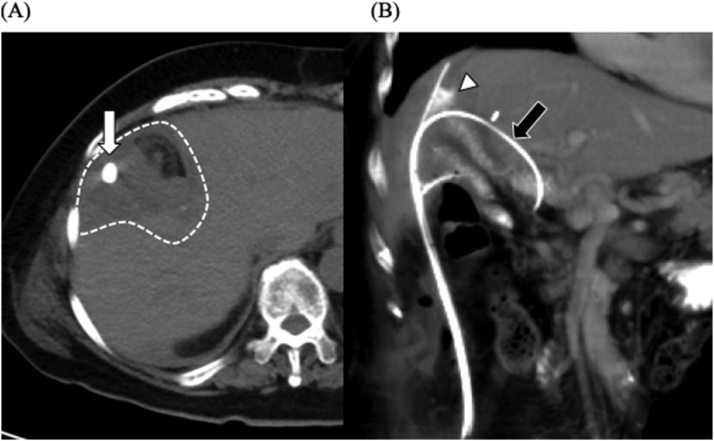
(A) Enhanced computed tomography (CT) on the 9^th^ postoperative day. The abscess cavity had formed around the surface of the hepatectomy from the bile duct injury point (dashed line), showing the indwelling drainage tube (white arrow). (B) Enhanced CT on the 20^th^ day after the intervention, showing the Cobra catheter in the bile duct (black arrow). The abscess cavity had almost disappeared (white arrowhead).
